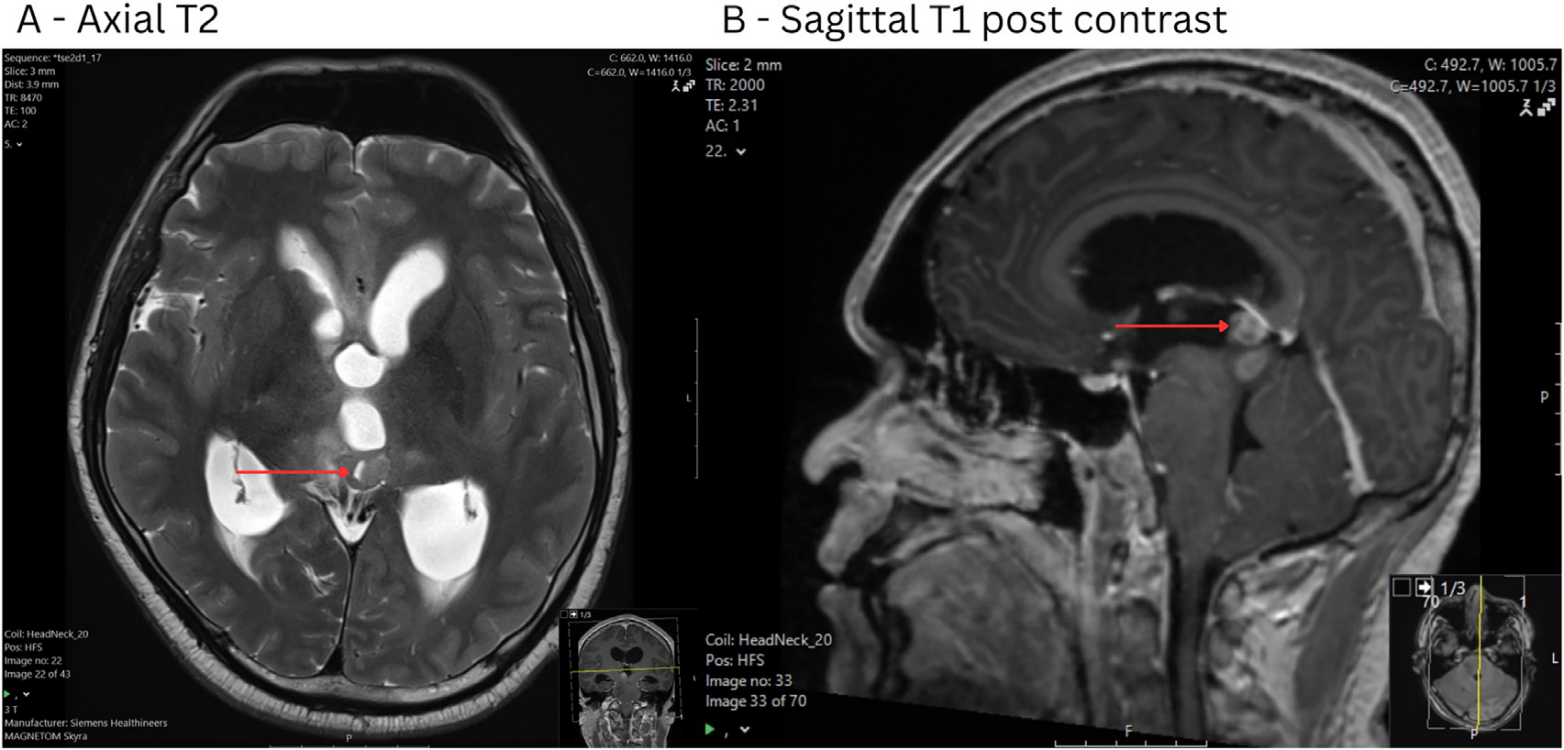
MRI images of a pineal gland lesion including: (A) Axial T2, (B) Sagittal T1 post contrast. This was taken at a later date and the patient developed hydrocephalus. 16 × 11 × 10 mm heterogeneously enhancing mass in the region of the pineal gland, of peripheral mild T2/FLAIR hyperintensity, T1 isointensity and central T2 hyperintensity/nonenhancement. Subtle associated diffusion restriction.
